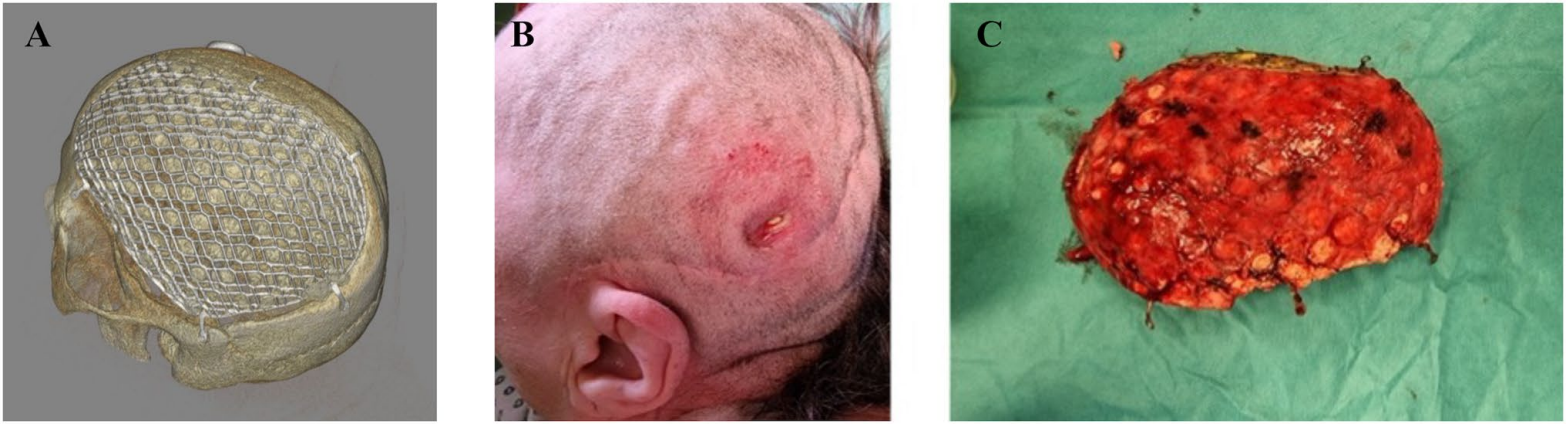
CT scans (A) prior to the explantation of a bioactive ceramic implant due to contaminated atrophic skin lesion from pathological head posture leading (B). No bacterial infection was detected on the implant itself. The implant showed signs of strong osseointegration with the surrounding tissue (C)
